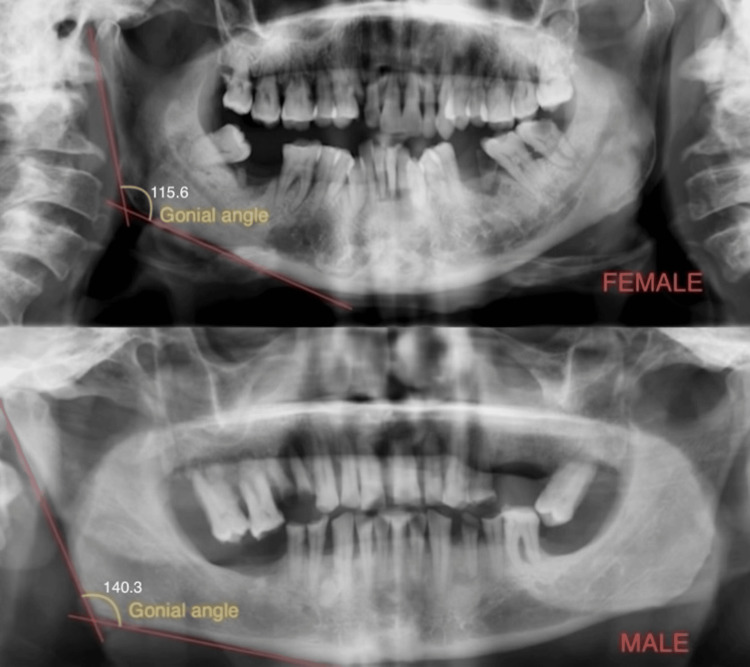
Representation of gonial angle in male and female (61 to 70 years) orthopantomogram.
